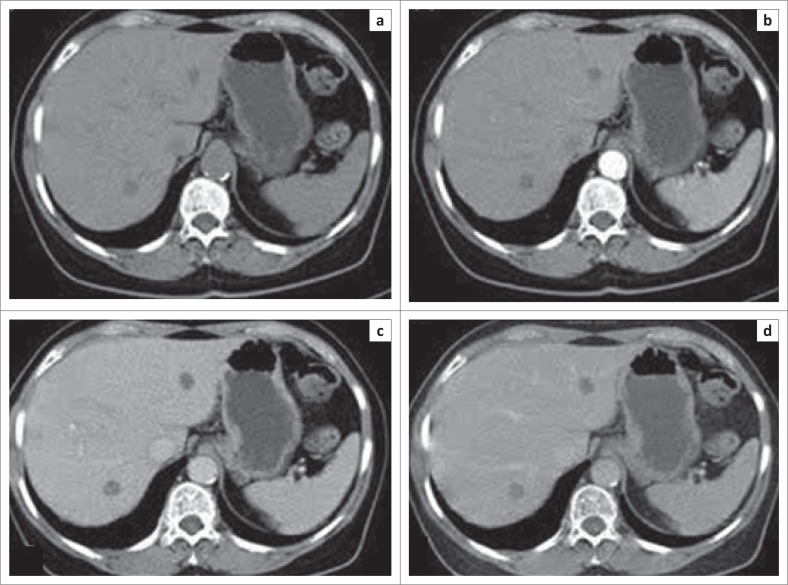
A 70-year-old man who presented with abdominal pain with no known primary malignancy. Axial computed tomography scan images of biopsy proven metastatic adenocarcinoma showing: (a) hypo-attenuation in the precontrast phase and no enhancement in (b) arterial, (c) portovenous and (d) delayed phase.
